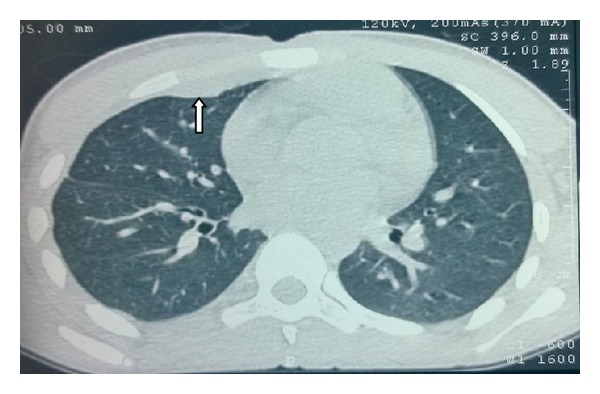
Axial CT image at lesion level in lung window showing no evidence of lung parenchymal involvement.
